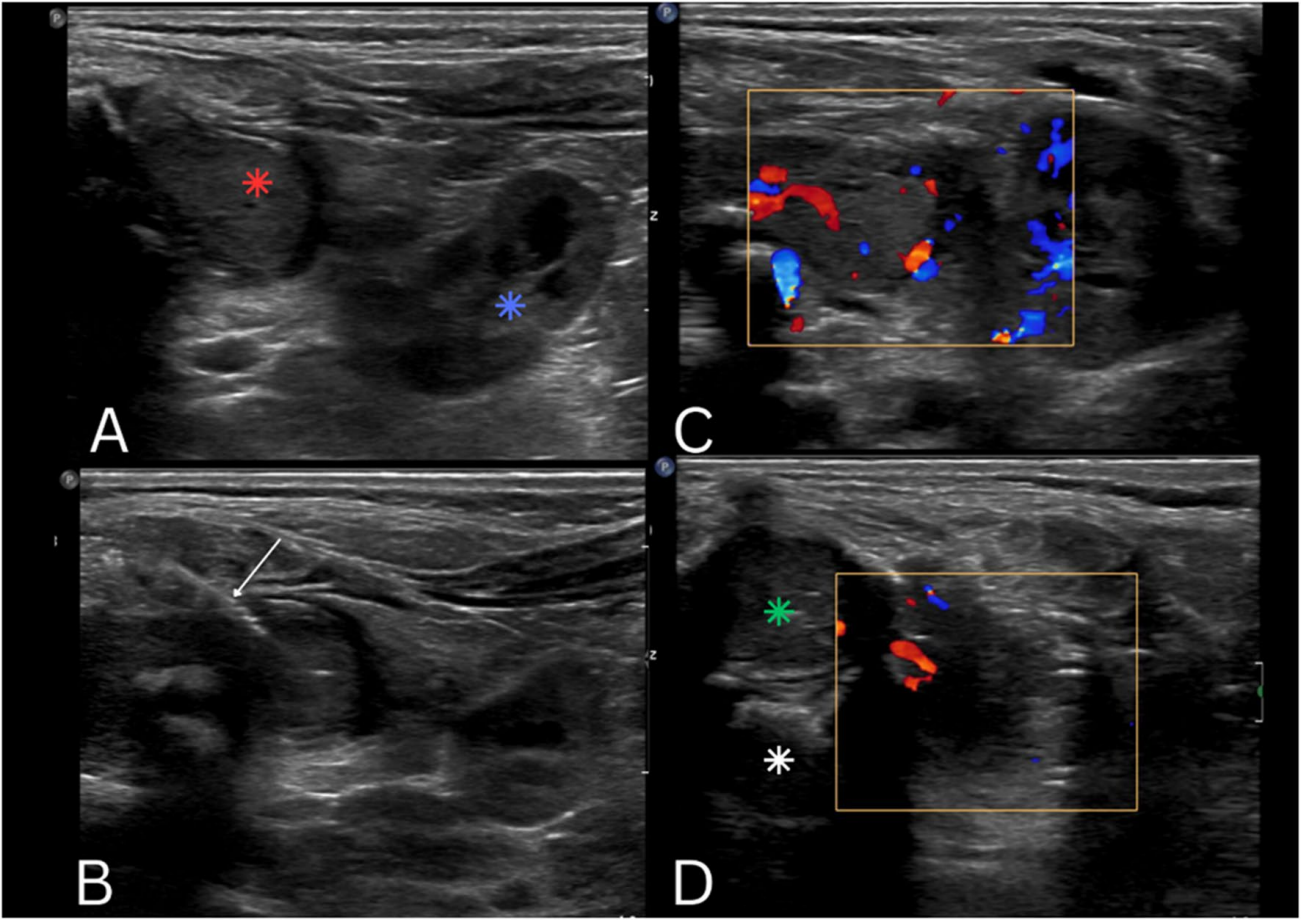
Transverse ultrasound images of the spine and right paraspinal region during transcutaneous biopsy. There is a hypoechoic structure occupying the pine canal (green asterisk, image D) with the vertebral body anteriority (white asterisk, image D) with a protruding component through the right neural foramina (red asterisk, image A) adjacent to the right kidney (blue asterisk, image A). The lesion shows increased flow on doppler images, comparable to that of the adjacent kidney (image B). Note is made of the biopsy needle (white arrow, image B)
